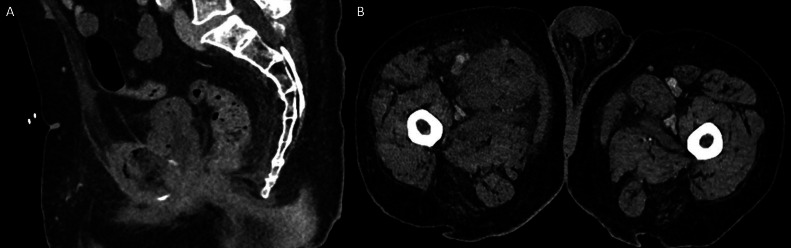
CT scan in the portal venous phase. (A) Sagittal slice showing a thick-walled urinary bladder adherent to the pubic symphysis with extensive surrounding fat stranding. Multiple gas locules are visible adjacent to the pubic symphysis. (B) Axial slice demonstrating inflammatory changes in the bilateral adductor compartments, more pronounced on the right, with a rim-enhancing collection within the right adductor longus and a smaller abscess in the adductor magnus.
